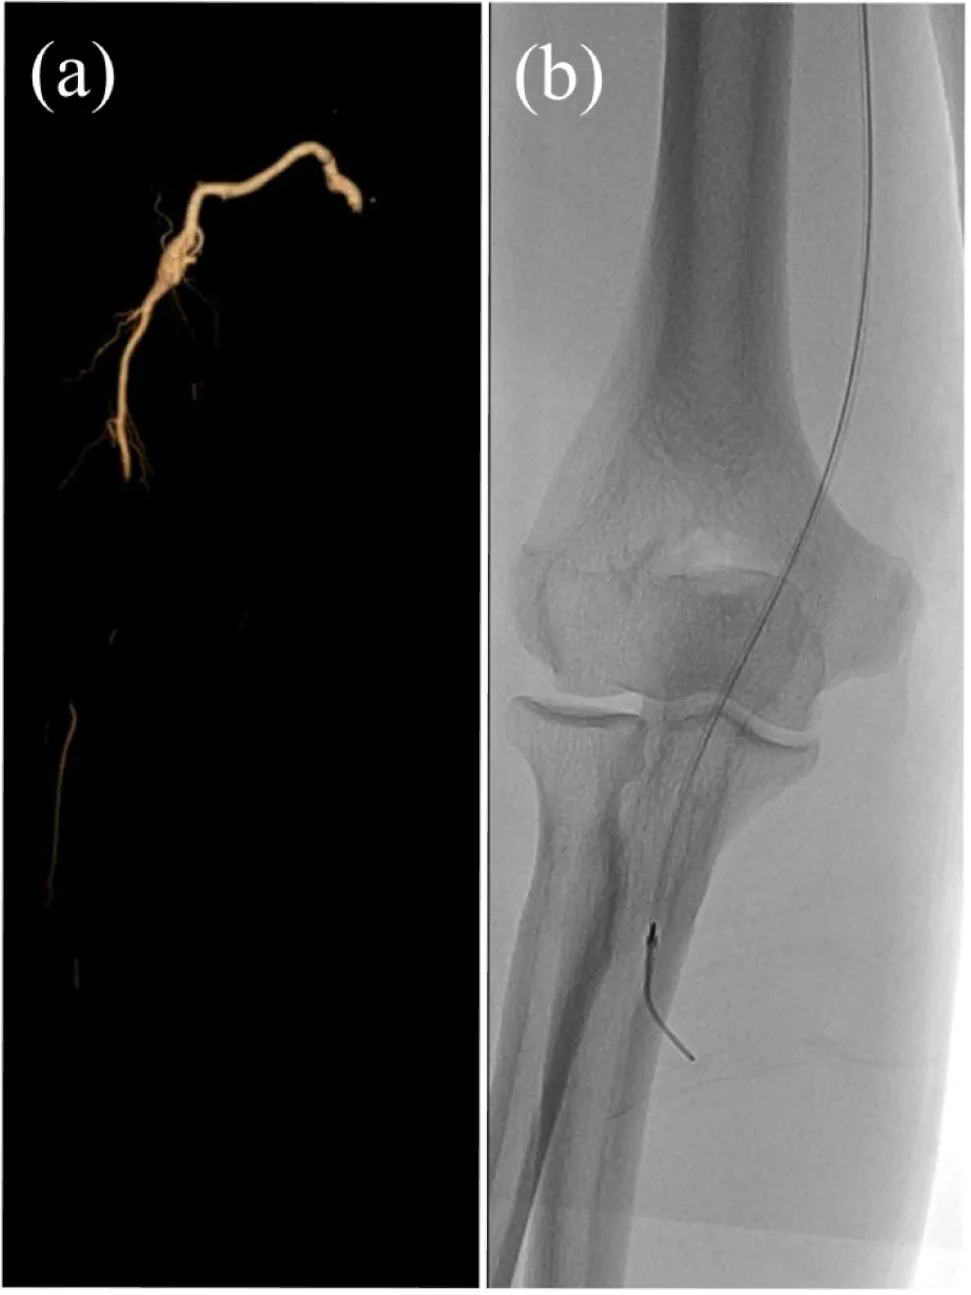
Emergency CTA and intraoperative fluoroscopy of the second intervention. (a) Emergency postoperative CTA after first thrombectomy: Residual mural thrombus within axillary aneurysm and partial re‐occlusion of distal limb arteries; (b) Intraoperative fluoroscopy: Catheter placement within brachial artery for directed thrombolysis.
